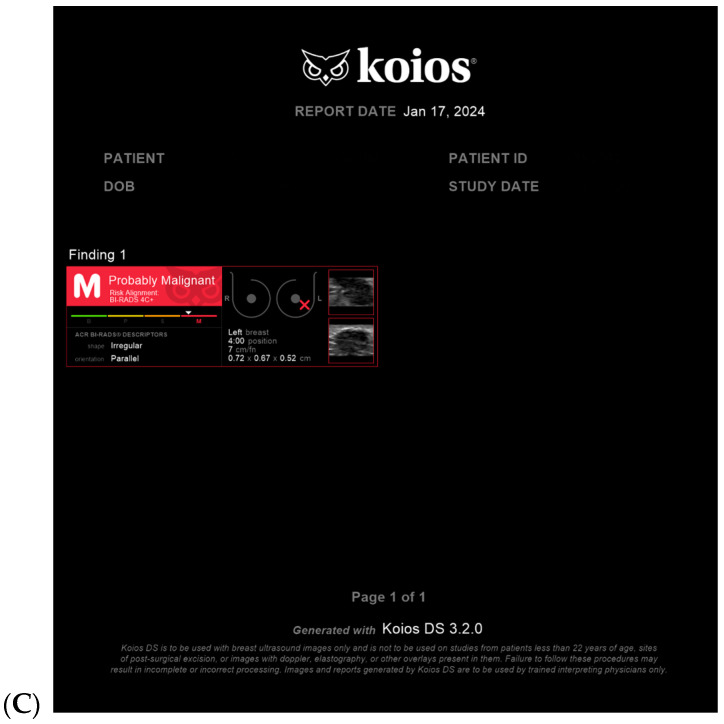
A new cancer diagnosis appropriately classified as “malignant” by artificial intelligence (AI): This patient in her 40s with a history of left breast carcinoma diagnosed 1 year prior, status post-left mastectomy with chemotherapy and hormonal therapy, presented with a palpable abnormality in the superficial lower outer left breast. No new or suspicious findings were seen on the patient’s diagnostic mammogram. Correlating with the patient’s concern about a palpable lump, diagnostic ultrasound revealed an irregularly shaped, hypoechoic mass with angular margins that are non-parallel (A), and Doppler shows no vascularity (B). The AI program Koios recognized this mass as “Probably Malignant” (C). This was returned as biopsy-proven invasive ductal carcinoma. Images obtained from the Icahn School of Medicine at Mount Sinai.
